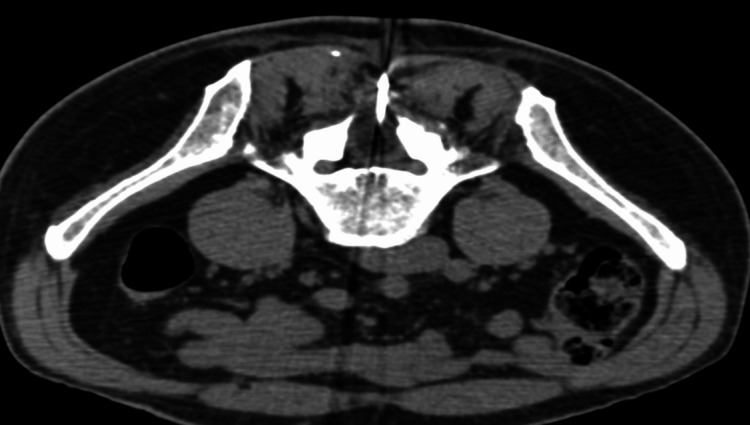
Axial image of the CT-guided cryoablation performed on June 3, 2023. The image demonstrates a cryoprobe inserted through the area of the prior lumbar laminectomy. The low-density oval-shaped area around the probe in the thecal canal represents the ice ball partially consuming the spinal tumor.
